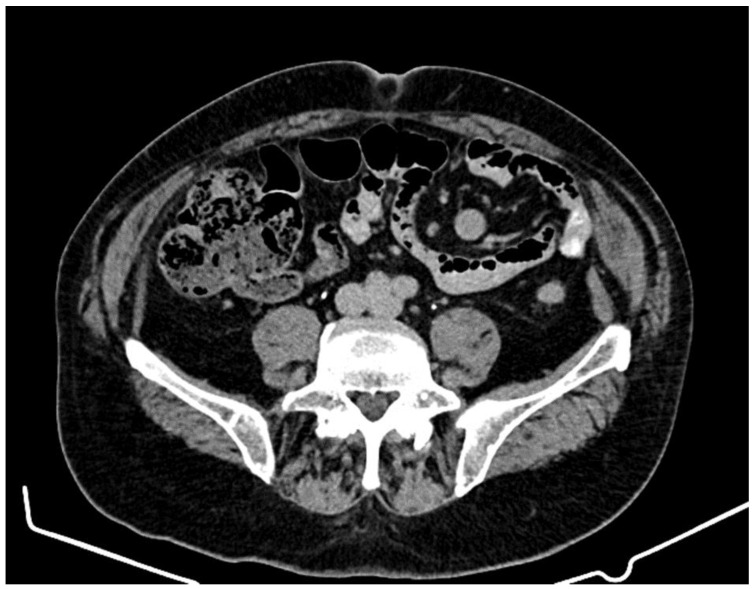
Computed tomography (April 2021): prostate with increased dimensions (axial diameters of 48/45 mm). The seminal vesicles presented no significant densitometry particularities.
